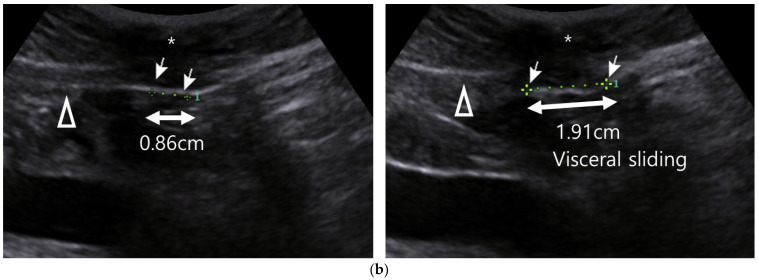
Visceral sliding technique via trans-abdominal ultrasound. Visceral sliding distances during tidal and maximal respiration are relatively shorter in cases with adhesions. (a) Visceral sliding during tidal and maximal respiration in a patient without adhesions; (b) visceral sliding during tidal and maximal respiration in a patient with adhesions. (*) Rectus abdominis muscle, (Δ) visceral peritoneum; Supplementary Video S1.
